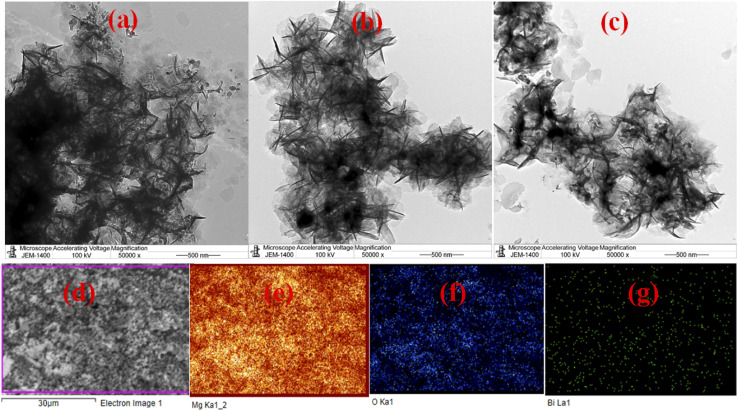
TEM images of (a) MgOBi1, (b) MgOBi2, and (c) MgOBi3, and. X-ray elemental mapping of (e) Mg−Ka, (f) O−Ka, and (g) Bi–La of the SEM MgOBi3 selected area (d).
